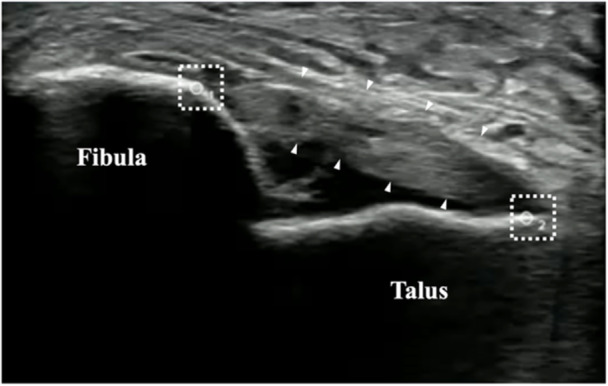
Ultrasonographic video imaging sites. ▲ anterior talofibular ligament (ATFL).
